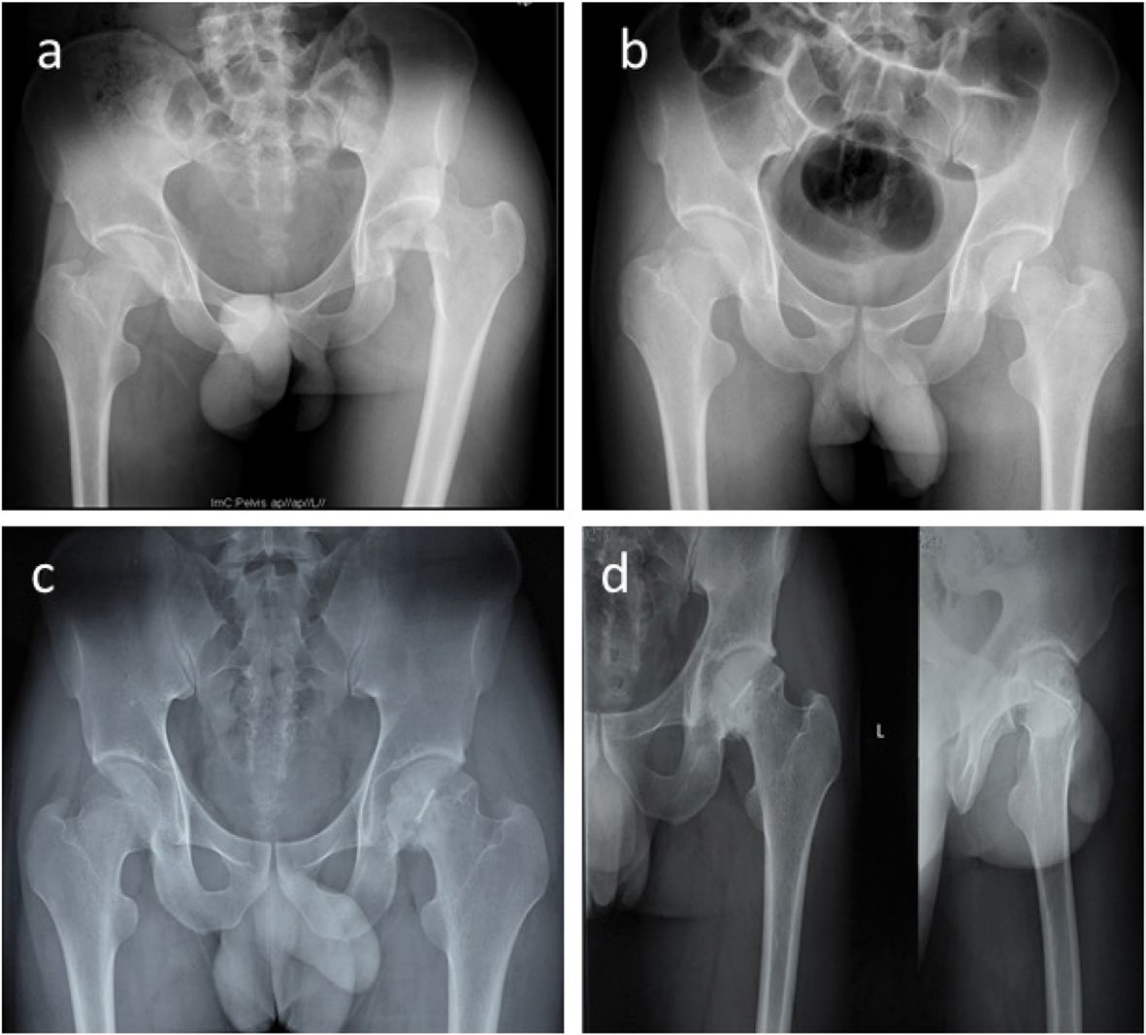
a Anteroposterior pelvic radiographs showing a 21-year-old male with left Pipkin II fracture-dislocation. b Post-operative X-ray films showing anatomical reduction of the femoral head with one Herbert screw via an anterior approach. c AP radiograph of 4th-year follow-up. d AP and Lateral radiograph of 7th-year follow-up.  There is evidence of post-traumatic osteoarthritis, including some loss of joint space, but there are no indications of AVN or heterotopic ossification
